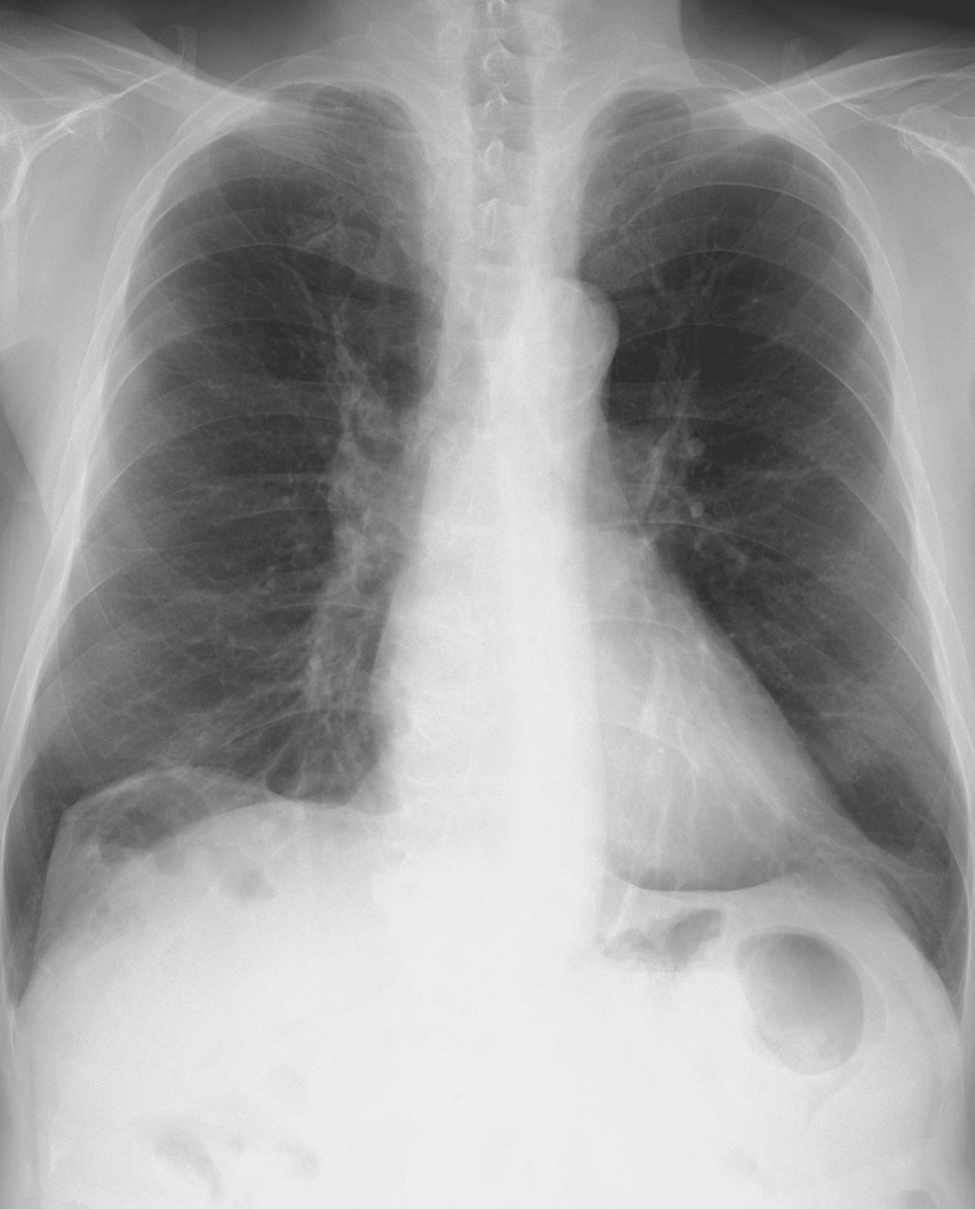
Chest x-ray showed hyperinflation of both lungs.
